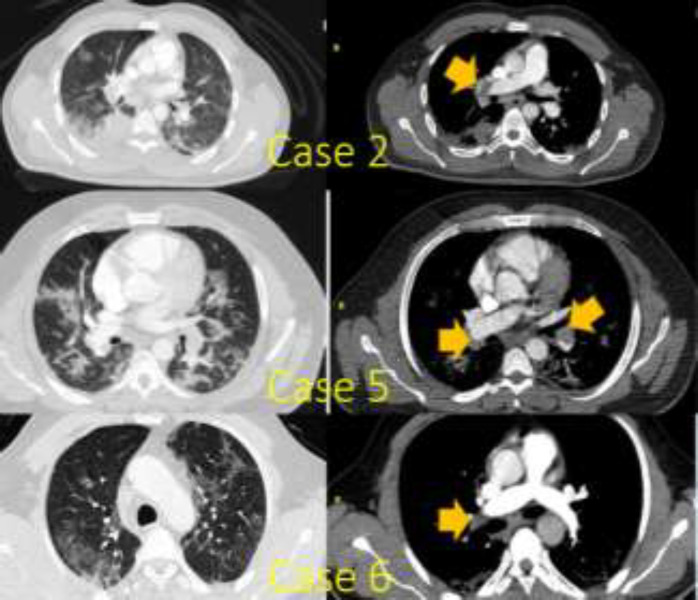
Lung CT scan and CT angiography findings in cases 2, 5, and 6
